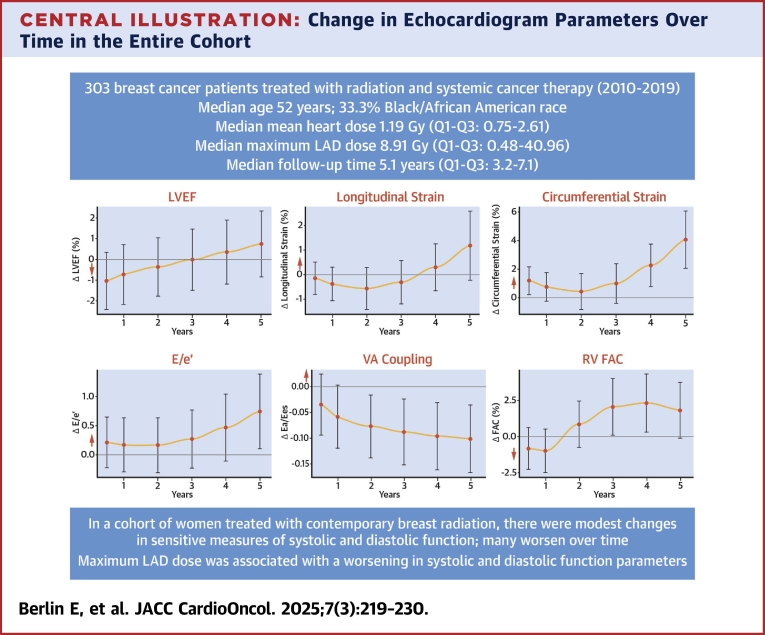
Change in Echocardiogram Parameters Over Time in the Entire Cohort Plots correspond to the predicted mean change in echocardiogram parameter over time from baseline (time = 0, pre–radiation therapy [pre-RT]) and at 0.5, 1, 2, 3, 4, and 5 years and were estimated by repeated-measures linear regression via generalized estimating equations. The model was adjusted for the following covariates: age, cancer stage (1 and 2 vs 3 and 4), body mass index, smoking status, systolic blood pressure, race, baseline hypertension, baseline diabetes, baseline cardioprotective medications (beta-blockers, angiotensin-converting enzyme inhibitors, angiotensin receptor blockers, statins), time since last chemotherapy exposure to start of radiation, systemic cancer therapy (anthracycline, trastuzumab, anthracycline + trastuzumab), time since initiation of radiation, treatment site (left/bilateral vs right), and baseline echocardiographic measure of interest. Lines within bars represent 95% CIs. Along the y-axis, a bold red arrow is placed to indicate the directionality of a detriment in cardiovascular function relative to baseline. The x-axis represents the years since radiation therapy initiation. E = early diastolic mitral inflow velocity; e′ = early diastolic mitral annular tissue velocity; Ea = systemic arterial load; Ees = left ventricular contractile function; FAC = fractional area change; LVEF = left ventricular ejection fraction; RT = radiation therapy; VA = ventricular-arterial.
